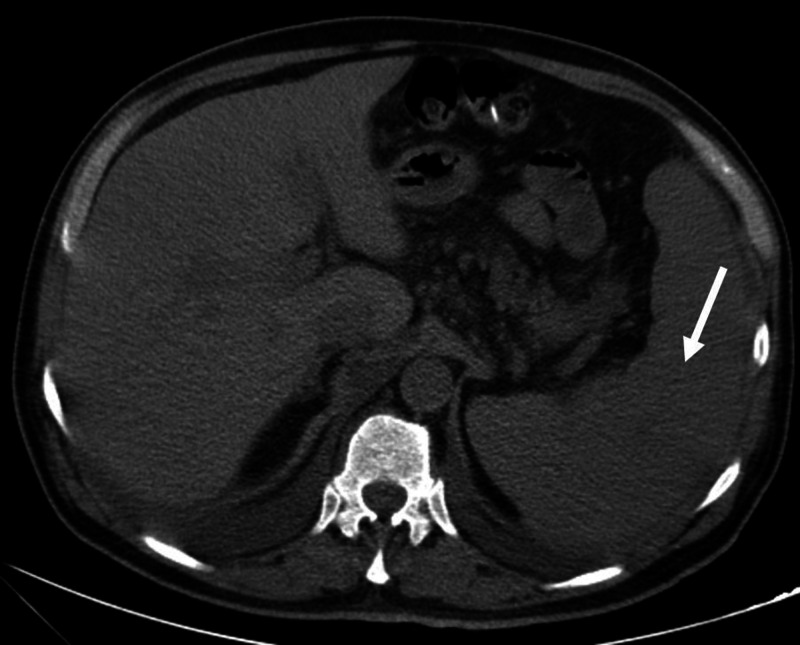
Computed tomography scan An enlarged spleen is seen (white arrow).
